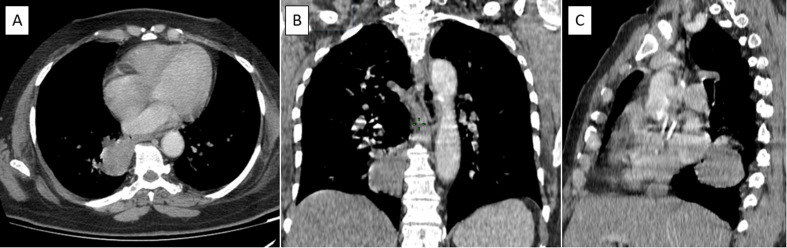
thoracic computed tomography demonstrating a right mediobasal pulmonary mass; axial (A); coronal (B); and sagittal (C) views
